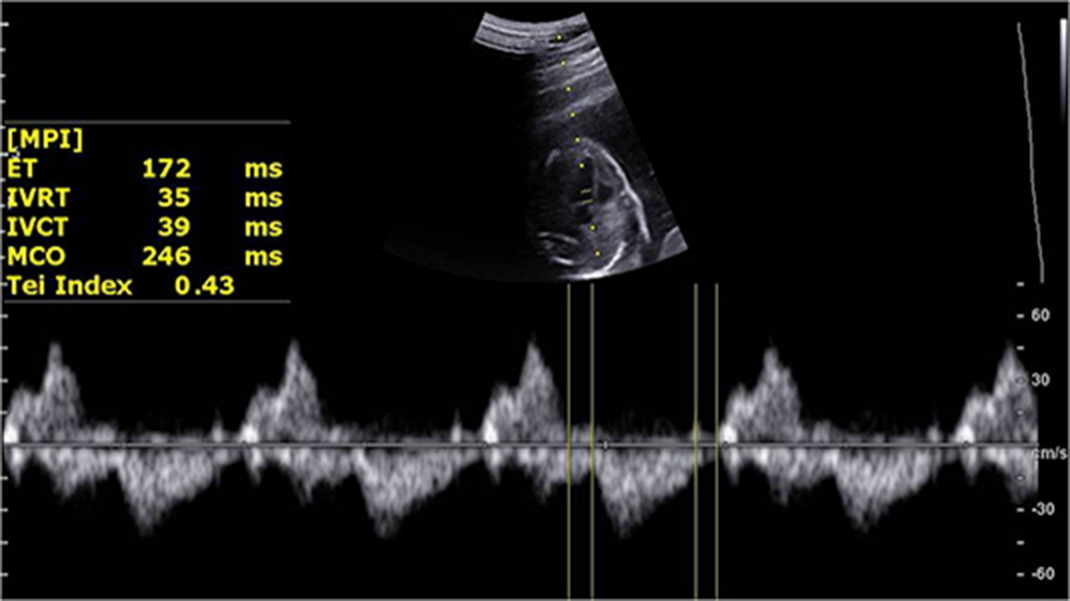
Normal Mod‐MPI (Tei index) Doppler image. The Doppler sample was located at the cross point between the inflow and outflow of the left ventricle, which included the leaflet of the mitral valve and aortic valve with a 3‐ to 4‐mm sample size. MCO, mitralvalve closing; and open time, ICT + IRT + ET.
